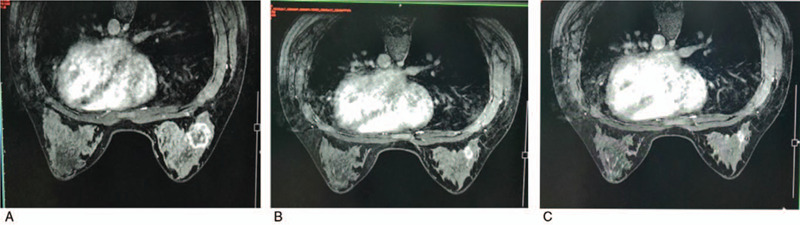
Evaluation of right breast mass by MRI during the neoadjuvant chemotherapy. (A) At the end of 4th cycle, a 3.1-cm breast mass was found; (B) at the 6th cycle, a 1.5-cm breast mass was found; (C) at the 8th cycle before operation, a 1.3-cm breast mass was found.
